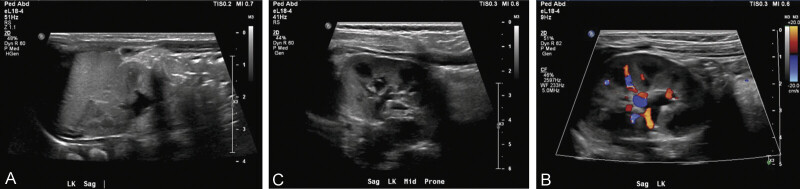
Renal ultrasound at approximately 6 months old.
